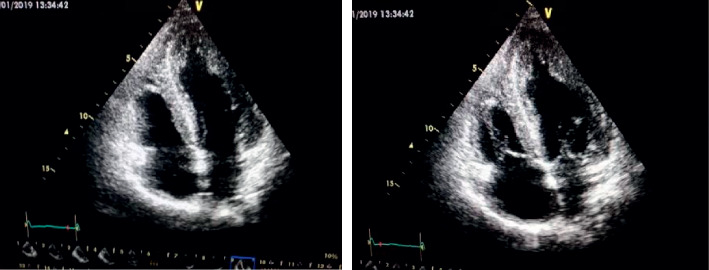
Echocardiogram photographs show granular sparkling of the myocardium walls suggesting cardiac amyloidosis.
